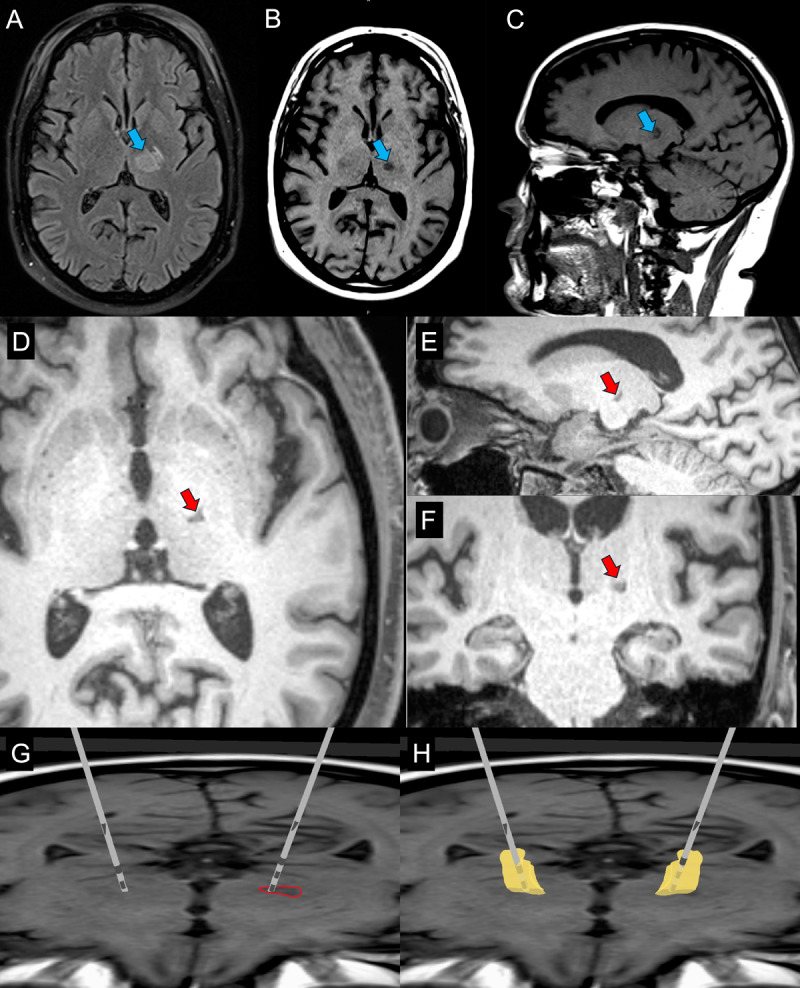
MRgFUS VIM Thalamotomy and VIM-DBS Localization. The MRgFUS lesion is visualized in the VIM thalamus on MRI on postoperative day 1 (blue arrows) demonstrating an adequate lesion on axial T2-FLAIR (A), axial T1-weighted (B) and sagittal T1-weighted (C) images. The lesion is redemonstrated at 24 months post-MRgFUS (red arrows) on T1-weighted MRI images in the axial (D), sagittal (E), and coronal (F) planes. Following DBS surgery, post-op CT was fused to pre-operative MRI, and the DBS lead was reconstructed using Lead-DBS [38]. The DBS lead is demonstrated within the previously lesioned tissue (red outline) on 24-month post-lesion axial T1-weighted MRI (H). The predicted VIM location (yellow), derived from the DISTAL atlas [39] and projected in MNI space, is superimposed on the same T1-weighted image (G).
